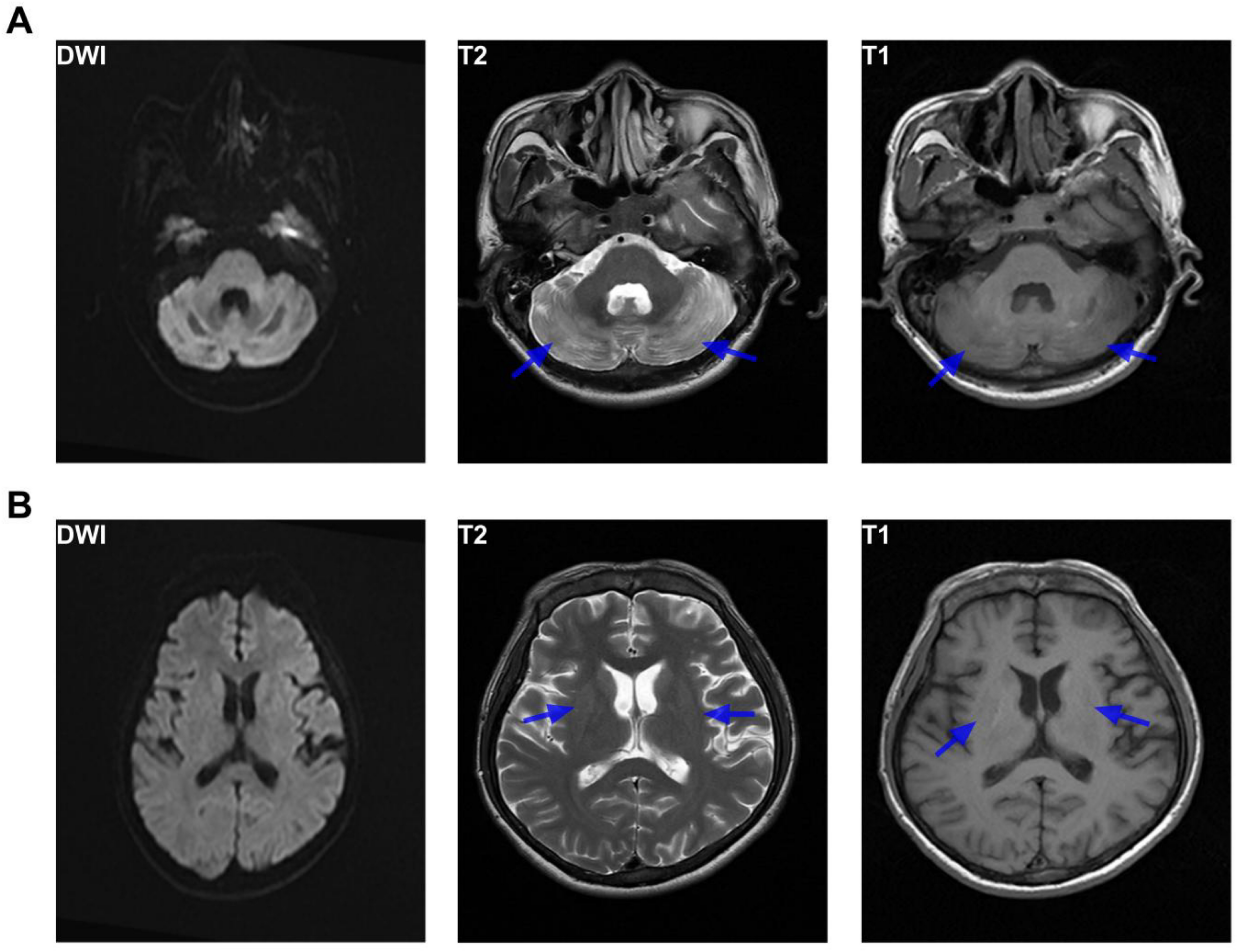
Brain MRI findings (day 51). Brain MRI showing (A) small patchy mixed T1- and T2-weighted signal abnormalities in the bilateral cerebellar hemispheres, with internal foci of short T1 signal, and (B) patchy mixed T1- and T2-weighted signal abnormalities in the bilateral basal ganglia with symmetrical distribution, as indicated by the blue arrows. DWI shows no obvious areas of high signal. Ventricular, cisternal, and sulcal morphology and positions are preserved, and midline structures are intact. The findings are consistent with typical imaging features of heatstroke. Compared with the MRI performed on 27 July 2022, the lesions show partial resolution and reduction in size.
